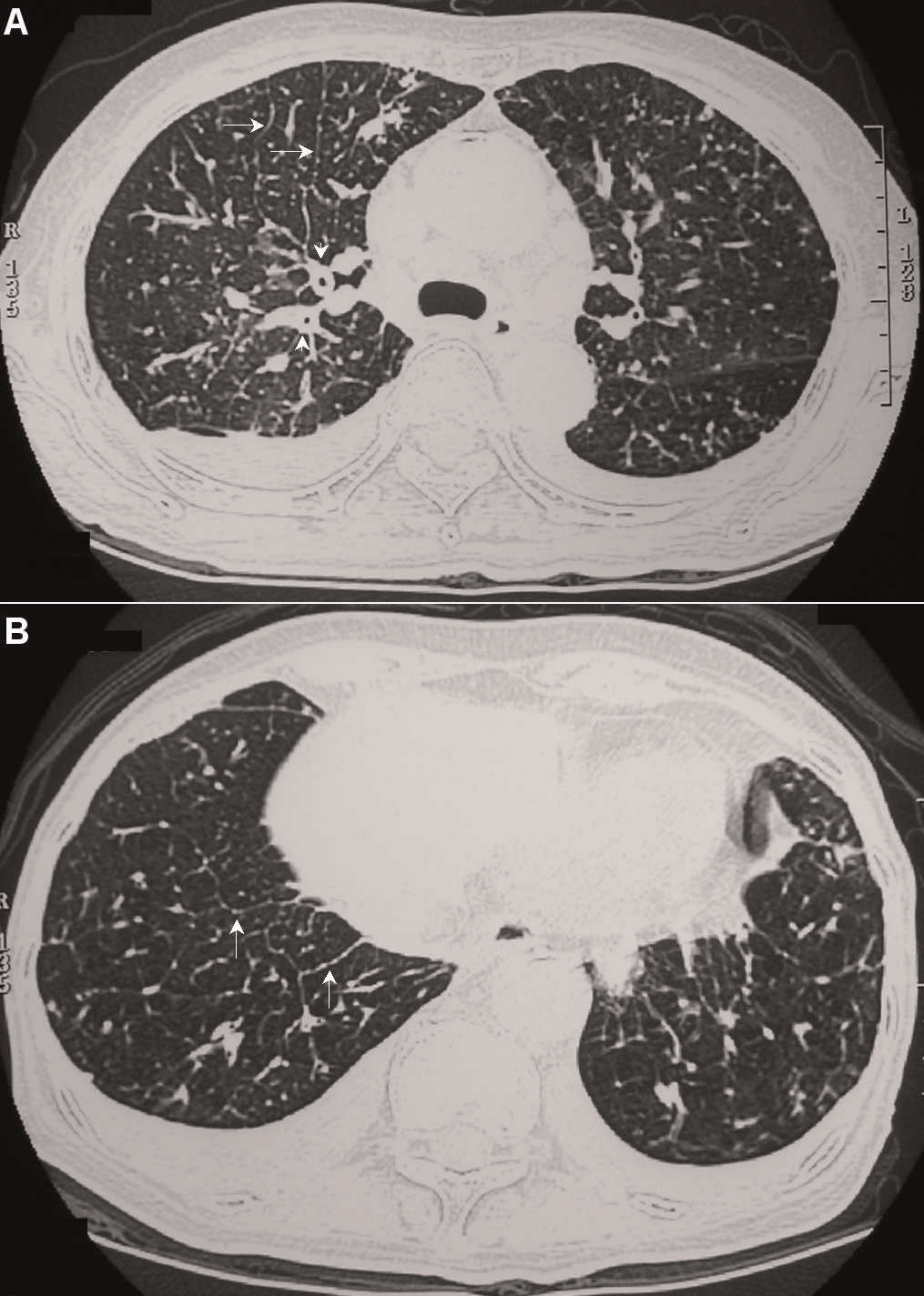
High-resolution chest computed tomography showing (A) Peribronchovascular thickening (arrowheads), irregular thickening of interlobular septa (arrows) and bilateral small amount pleural effusion (B) Interlobular septal thickening forming polygonal arcades (arrow) with prominence of the centrilobular bronchovascular bundle (a central dot in a polygon).
